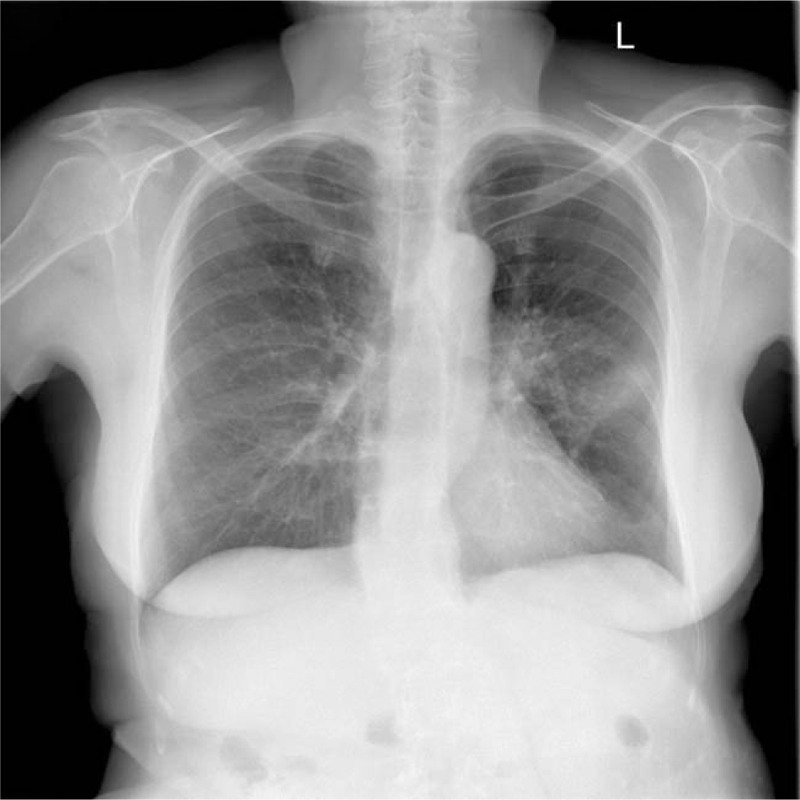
X-ray of the patient's lungs before the surgery, showing perihilar density with a visible air bronchogram in the middle field of the left lung.
